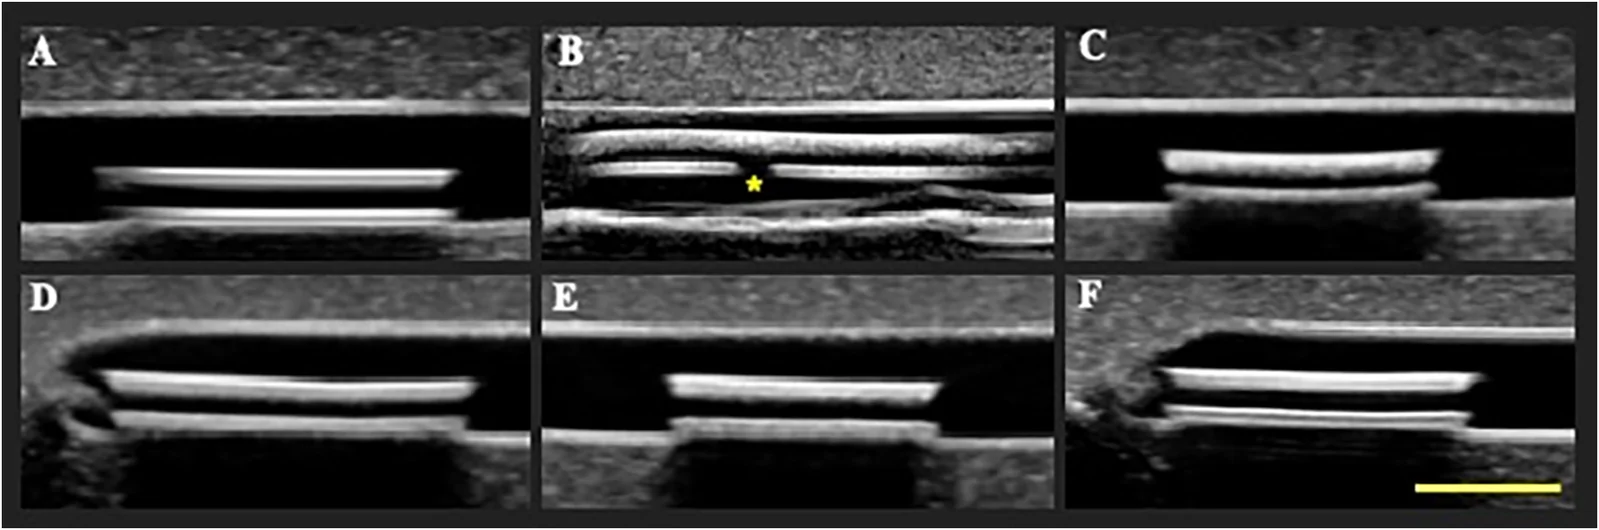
Representative sonograms of extruded tubes embedded in soft tissue
